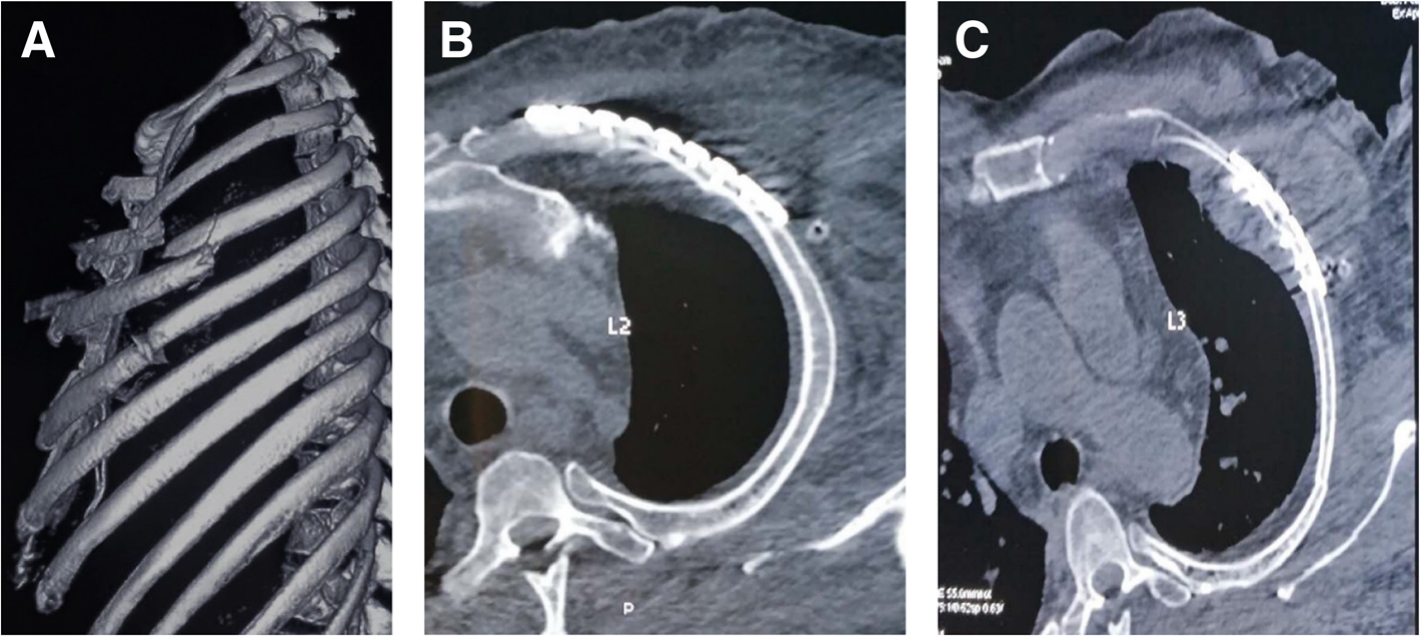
No.5 patient (a) The 3D model of the fracture end of the rib was adjusted by using 3D software model. b, c The postoperative CT locking plate position and thoracic morphology were great
